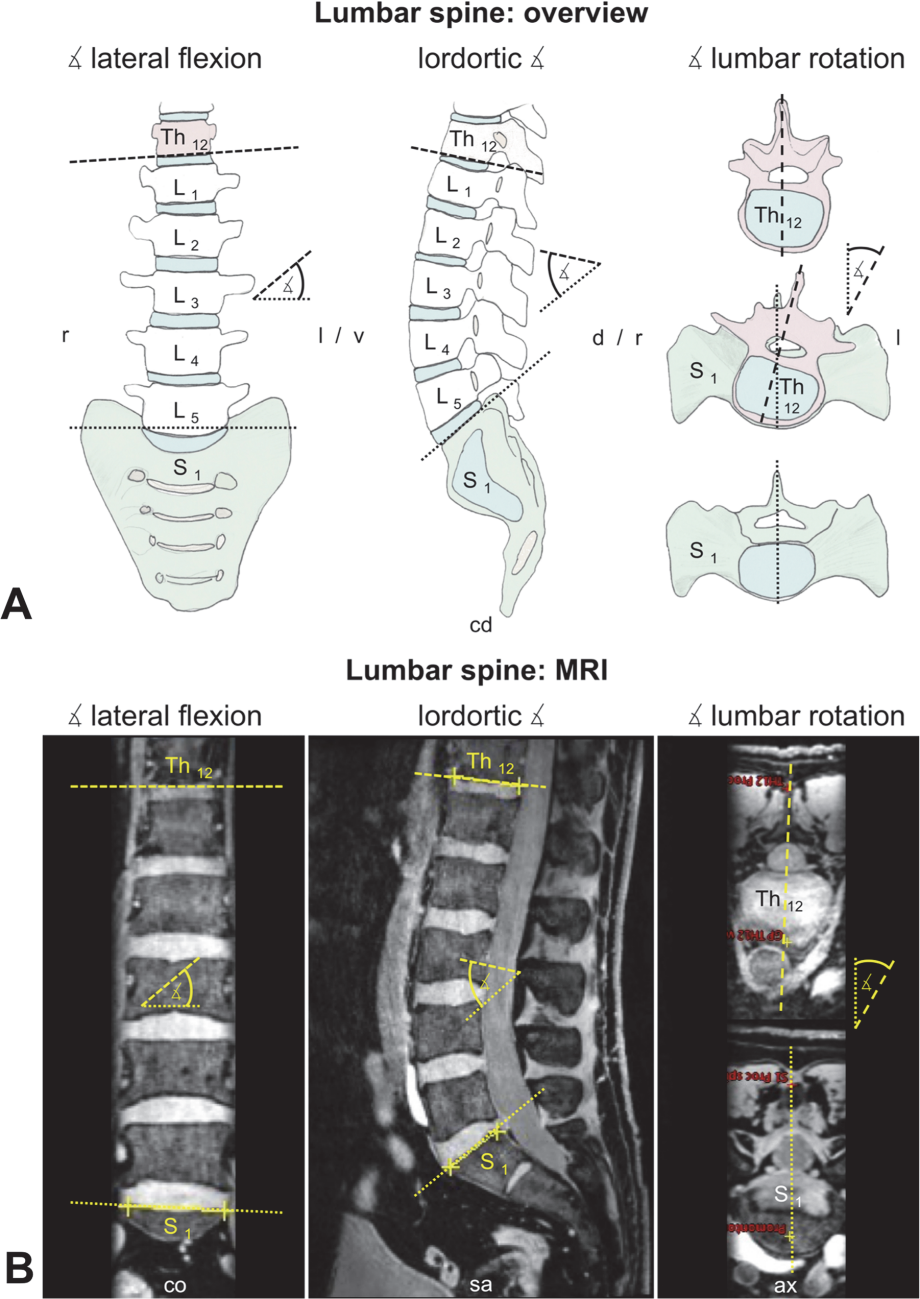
Morphometrical measurements. On basis of 3 Tesla magnetic resonance imaging, angles and spatial relations were compared at the lumbar spine. ASIS = anterior superior iliac spine, ax = axial plane, co = coronal plane, cd = caudal, cr = cranial, d = dorsal, l = left, MRI = magnetic resonance imaging, PSIS = posterior superior iliac spine, r = right, S _1,2,etc._ = first (second, etc.) sacral vertebral body, sa = sagittal plane, Th _12_ = twelfth thoracic vertebral body, v = ventral, ∡ = angle.
